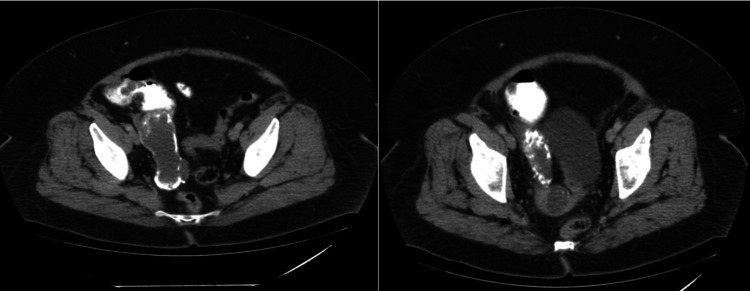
CT scan showing a markedly dilated, predominantly fluid-filled appendix consistent with a mucinous neoplasm.
